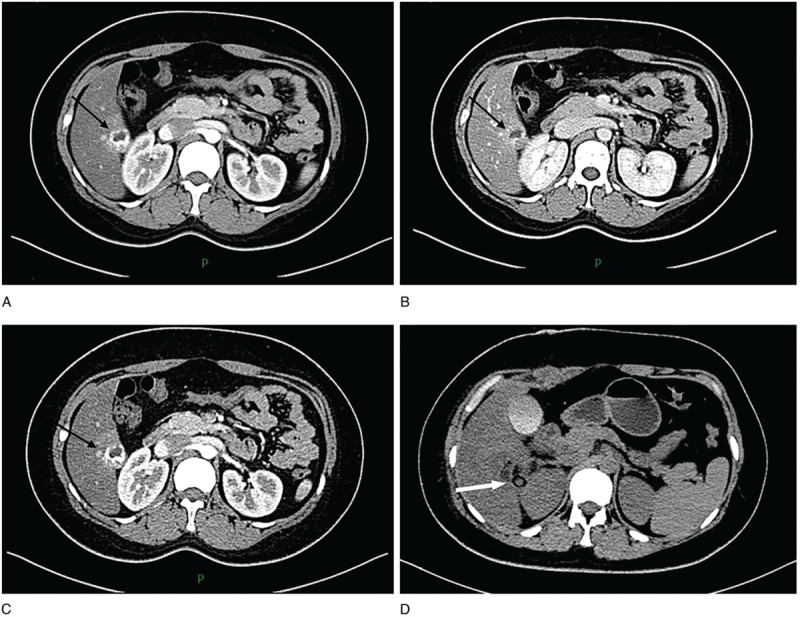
(A–C) Abdominal computed tomography performed with contrast enhancement. The arterial phase revealed a round lesion, with a peripheral portion hypervascularized with significantly increased density. The main axis of the lesion was 29 mm (black arrow). (D) Abdominal computed tomography scan showed the drainage tube drawn from the surgical field after the surgery (white arrow).
